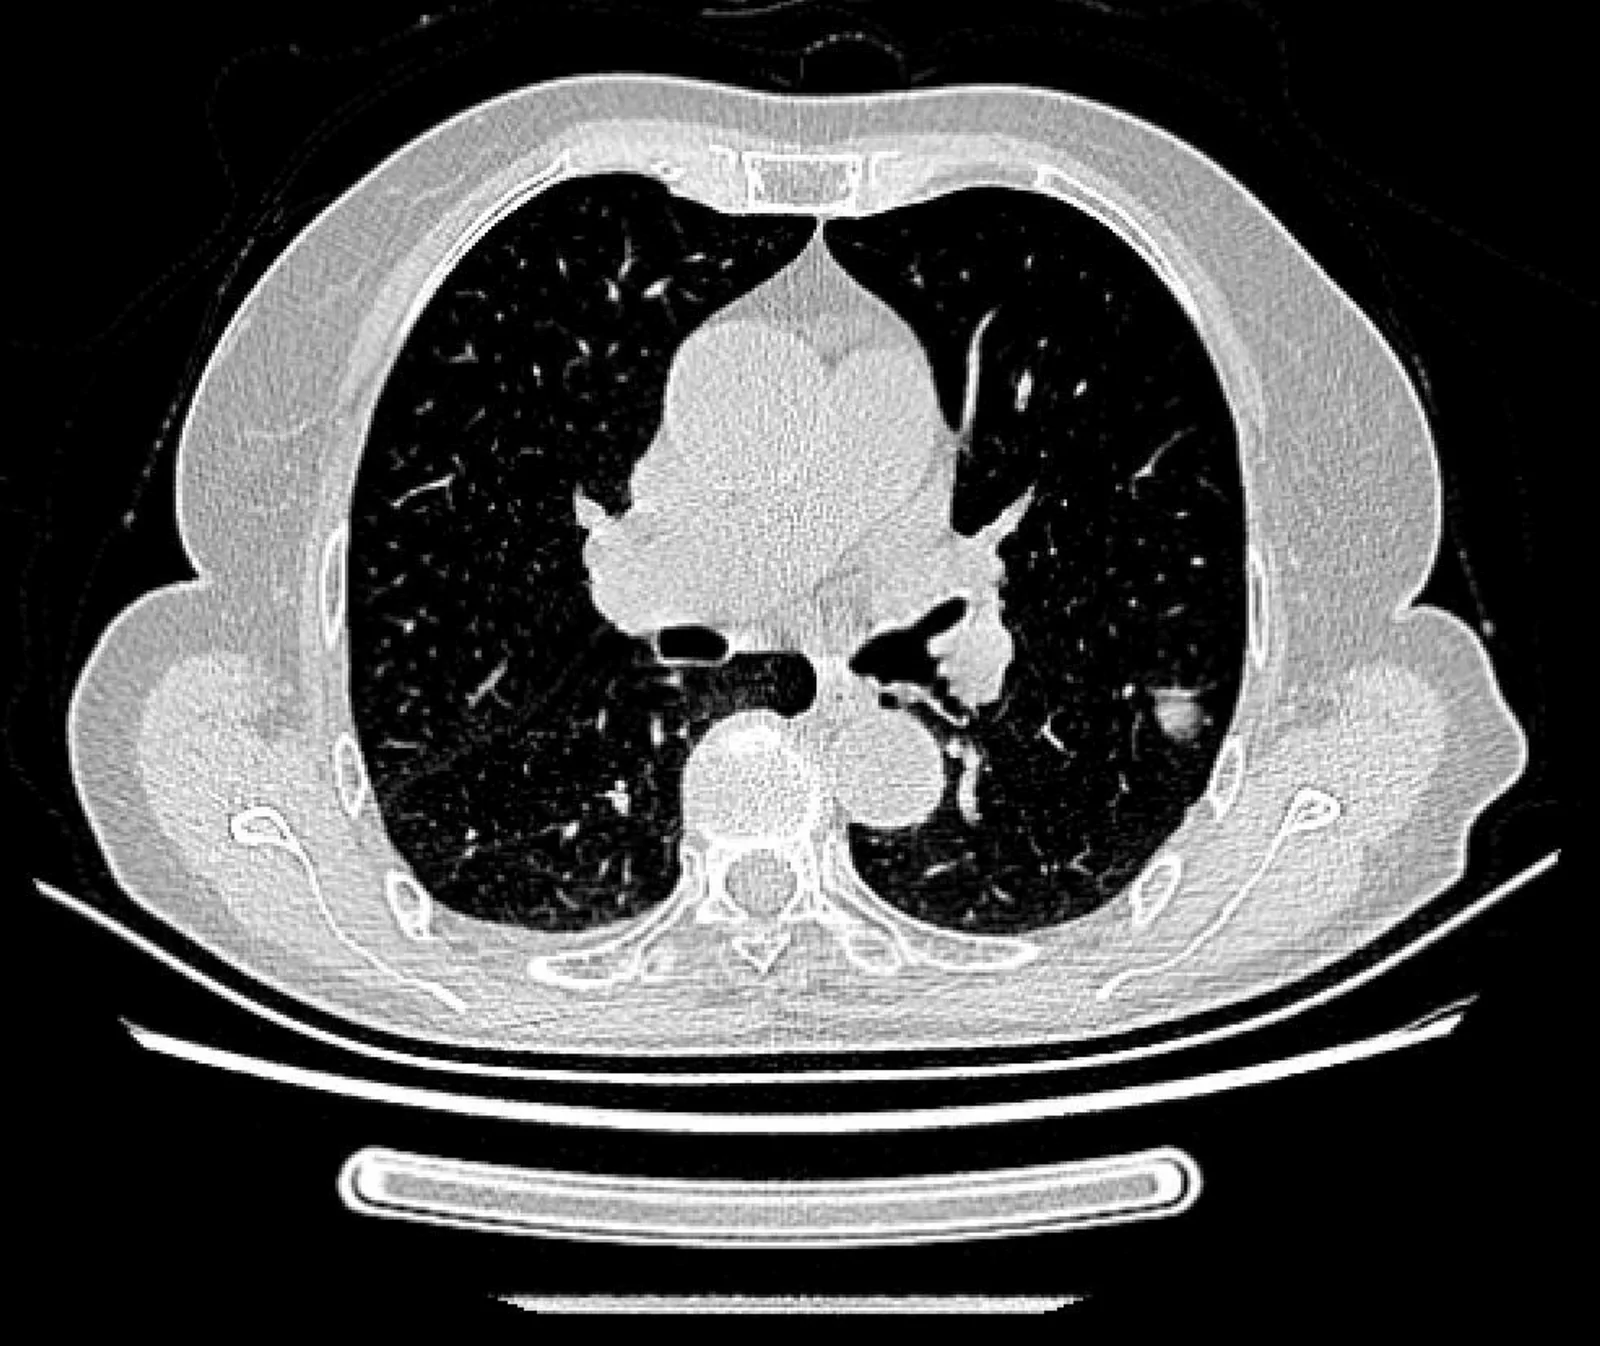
Noncontrast Chest Computed Tomography After Bronchoscopic Resection (Lung Window). The left upper-lobe bronchus is patent, with no definite residual endoluminal nodule.
